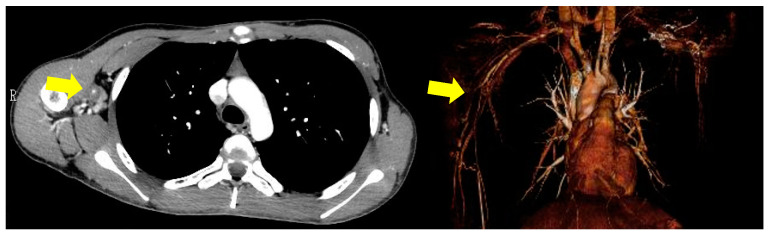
Contrast-enhanced CT images at the time of diagnosis of Takayasu’s disease. The yellow arrow showed a narrowing of the brachial artery.
